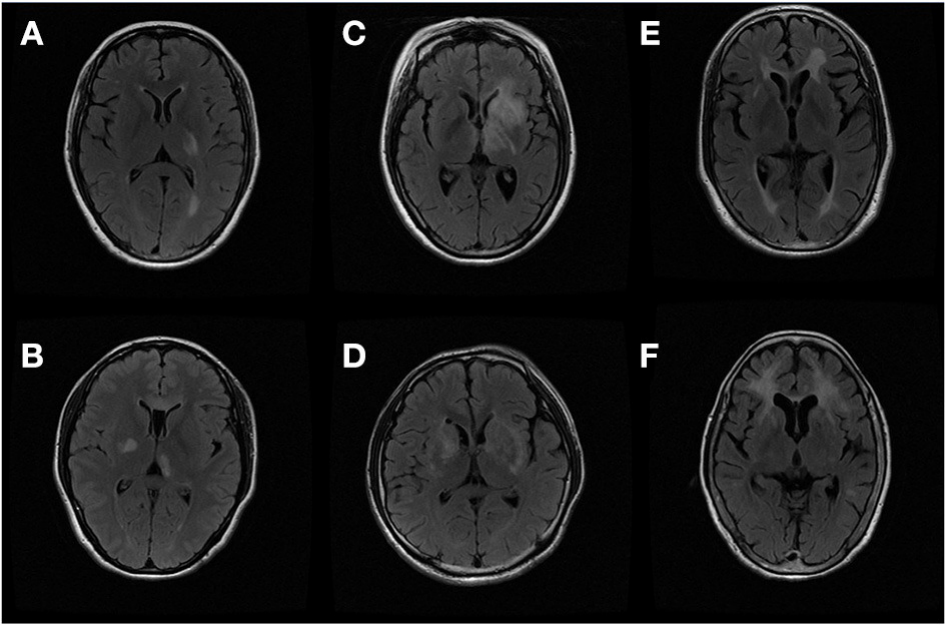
Thalamus and basal ganglia lesions on magnetic resonance image (MRI): (A,B) thalamus lesions of multiple sclerosis (MS) and primary central nervous system angiitis (PACNS); (C,D) internal capsule lesions of MS and PACNS; (E,F) putamen lesions of MS and PACNS.
